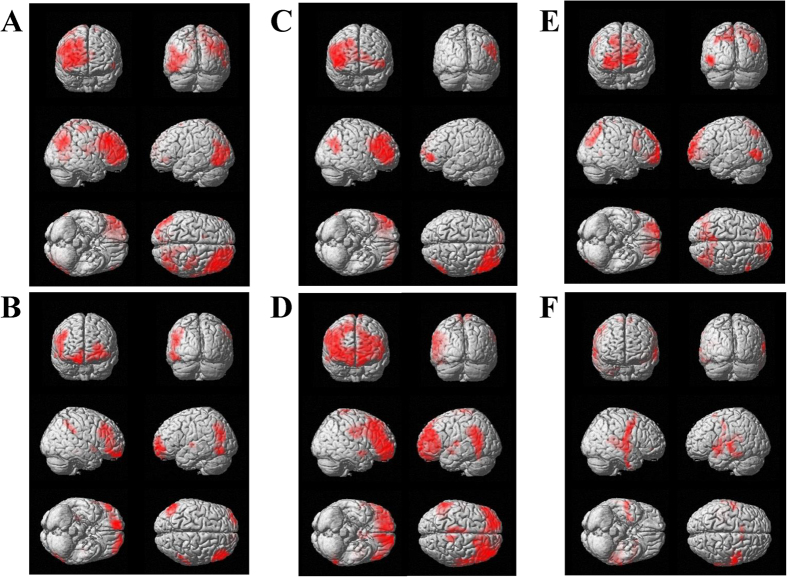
Statistical parametric maps of brain regions where decreases of oscillatory band power were observed in the prediction and control conditions. The decrease in alpha (8–13 Hz) band power observed in the time window of 1200 to 1350 ms in the prediction (A) and control (B) conditions, that observed in the time window of 1350 to 1500 ms in the prediction (C) and control (D) conditions, and the decrease in gamma (25–58 Hz) band power in the time window of 1500 to 1650 ms in the prediction (E) and control (F) conditions are shown. Statistical parametric maps are superimposed on surface-rendered high-resolution MR images. Random-effect analyses of 16 participants, familywise-error corrected for the entire search volumes. For the presentation purpose, the statistical threshold for (A–D) are set at *P* < 1.0 × 10^−6^ and that for (E,F) are set at *P* < 1.0 × 10^−5^.
